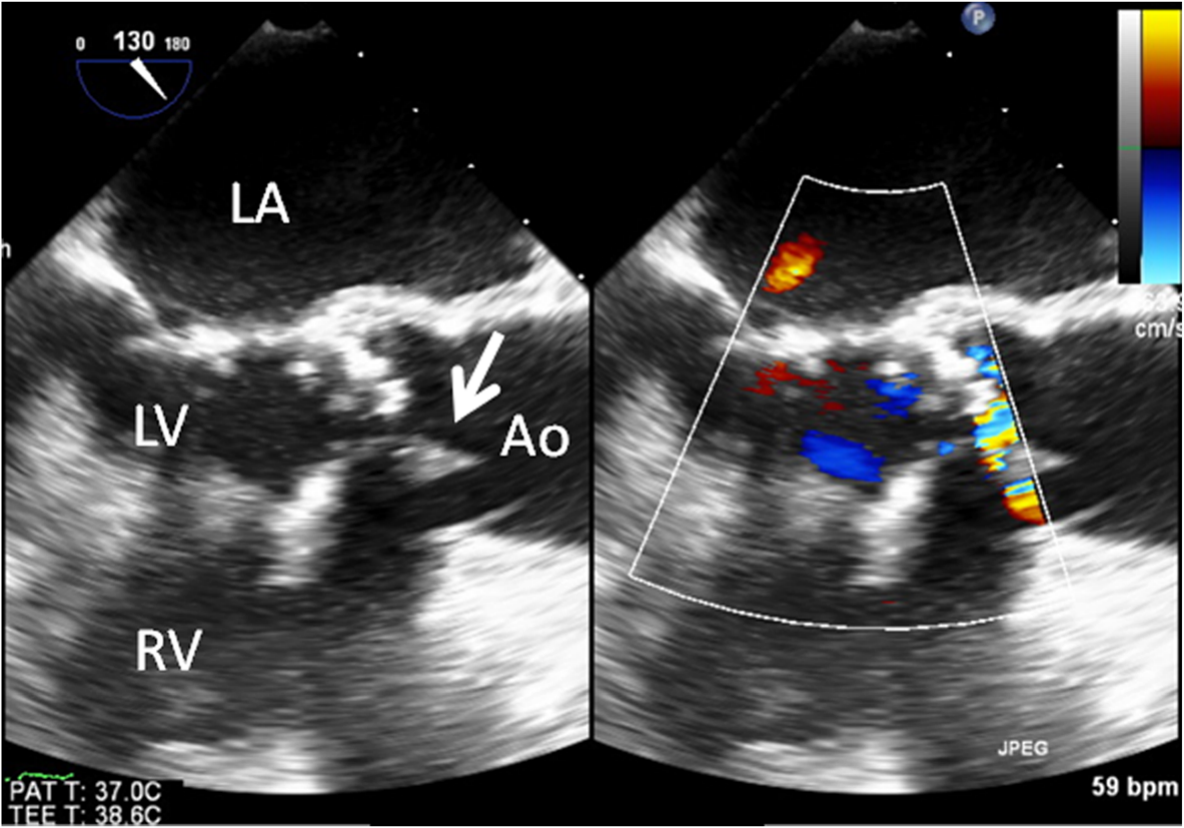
Transesophageal echocardiogram. A 12-mm diameter verruca was observed in the aortic valve at the non-coronary cusp-right coronary cusp (NCC–RCC) junction.
